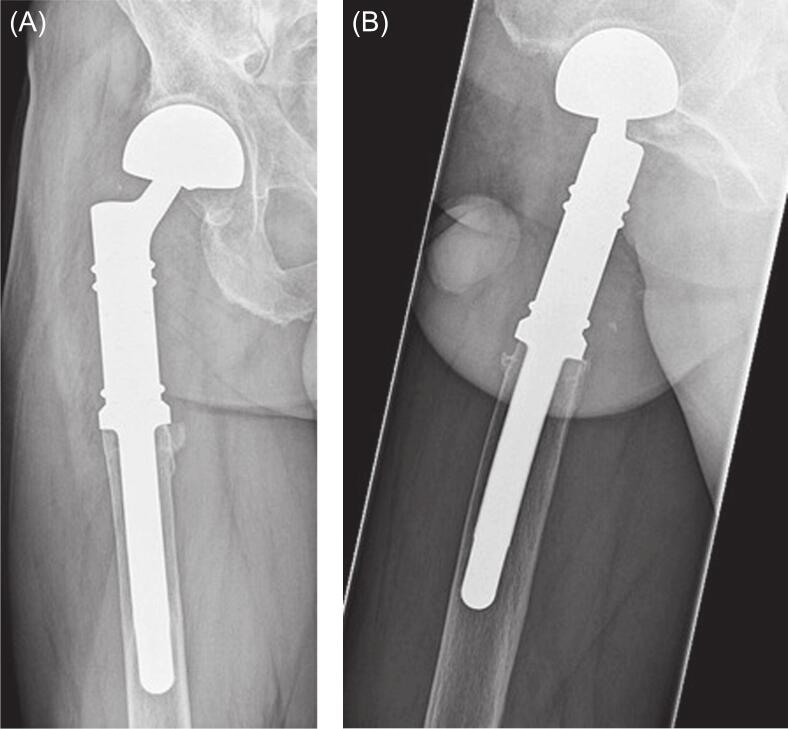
Conventional anteroposterior (1 A) and lateral (1B) radiographs, taken four years after resection of 12 cm of the proximal femur for a grade 2 chondrosarcoma. The defect was reconstructed with an uncemented hydroxyapatite-coated MUTARS® proximal femoral replacement with a bipolar femoral head and an attachment tube. At review, the patient was free from complications and functioned well.
